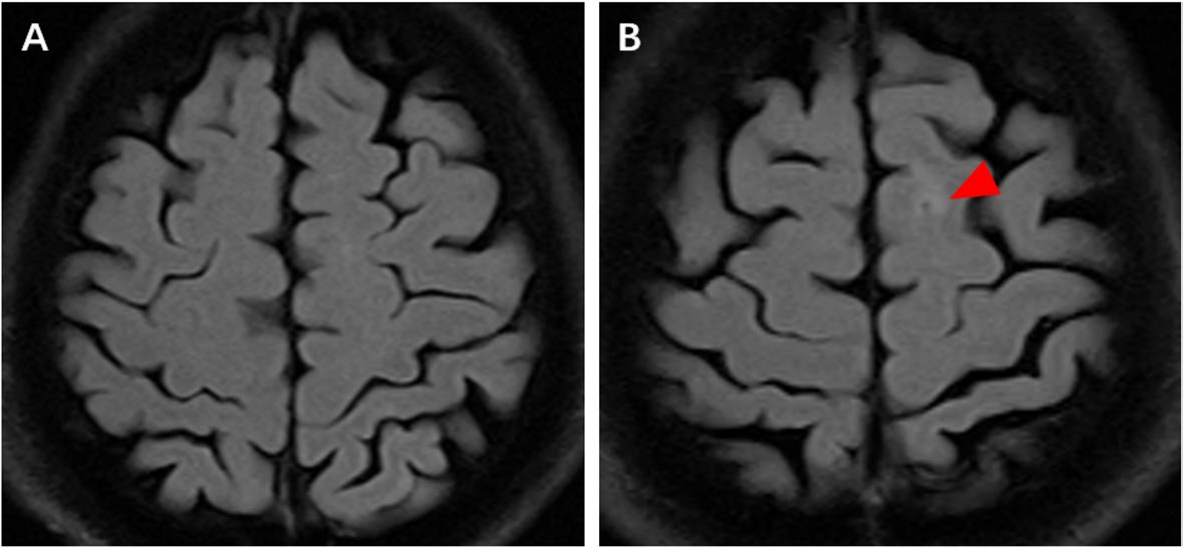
Postoperative brain MRI. A Absence of acute infarct lesions on postoperative brain MRI. B A probable microembolisation lesion in the left frontal lobe on brain MRI 7 days postoperatively (red arrow). MRI = magnetic resonance imaging
